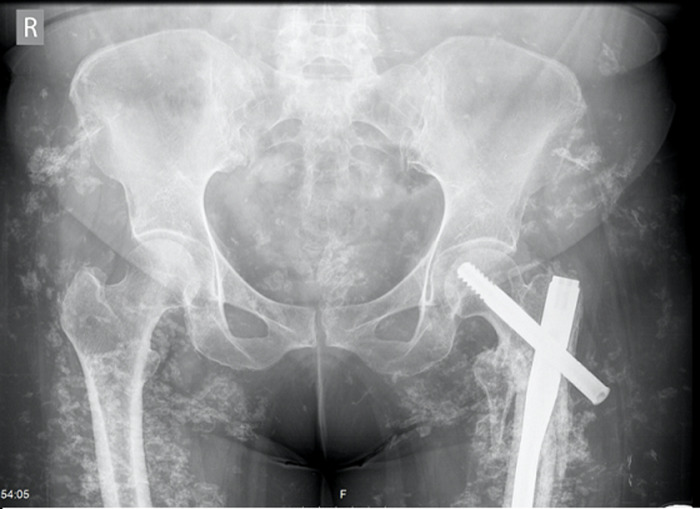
pelvic X-ray showing extensive soft tissue calcification
